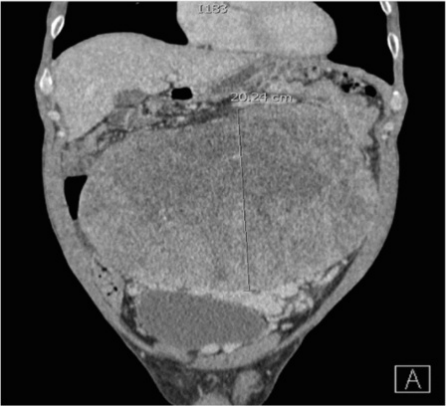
Coronal section computed tomography scan with intravenous contrast showing a large mass abutting the urinary bladder with engorged pelvic veins.
